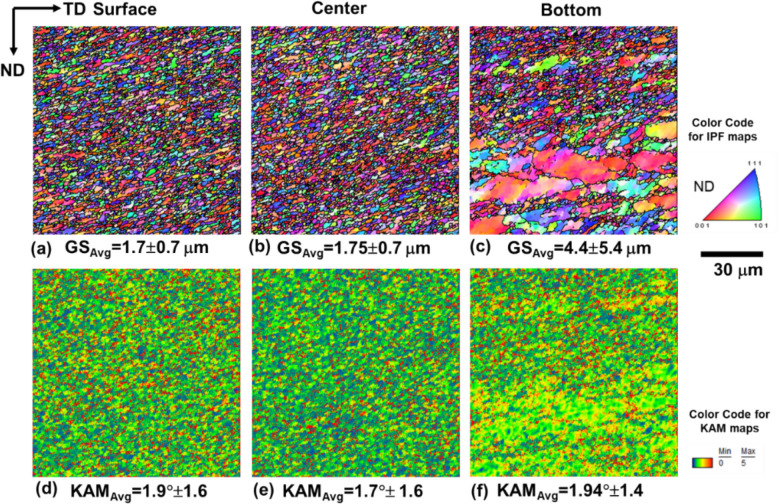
Through-thickness microstructural evolution in the FSPed region. a-c ND-IPF (inverse pole figure) maps and d-f Kernel average misorientation (KAM) maps for the surface, center, and bottom regions of the 600 RPM CT-FSPed sample. Note: Average grain size (GS_Avg_) was calculated considering 5° grain tolerance angle. KAM was calculated using the 3rd nearest neighbor. The surface and center correspond to the SZ, whereas the bottom lies near the SZ and TMAZ interface
